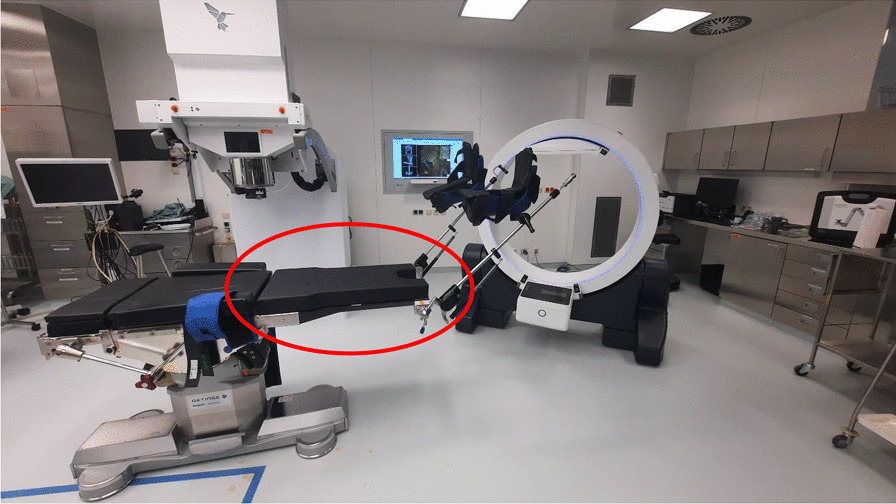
Carefully selected operating table setup with fully X-rays capable part between table Column and leg positioning device
